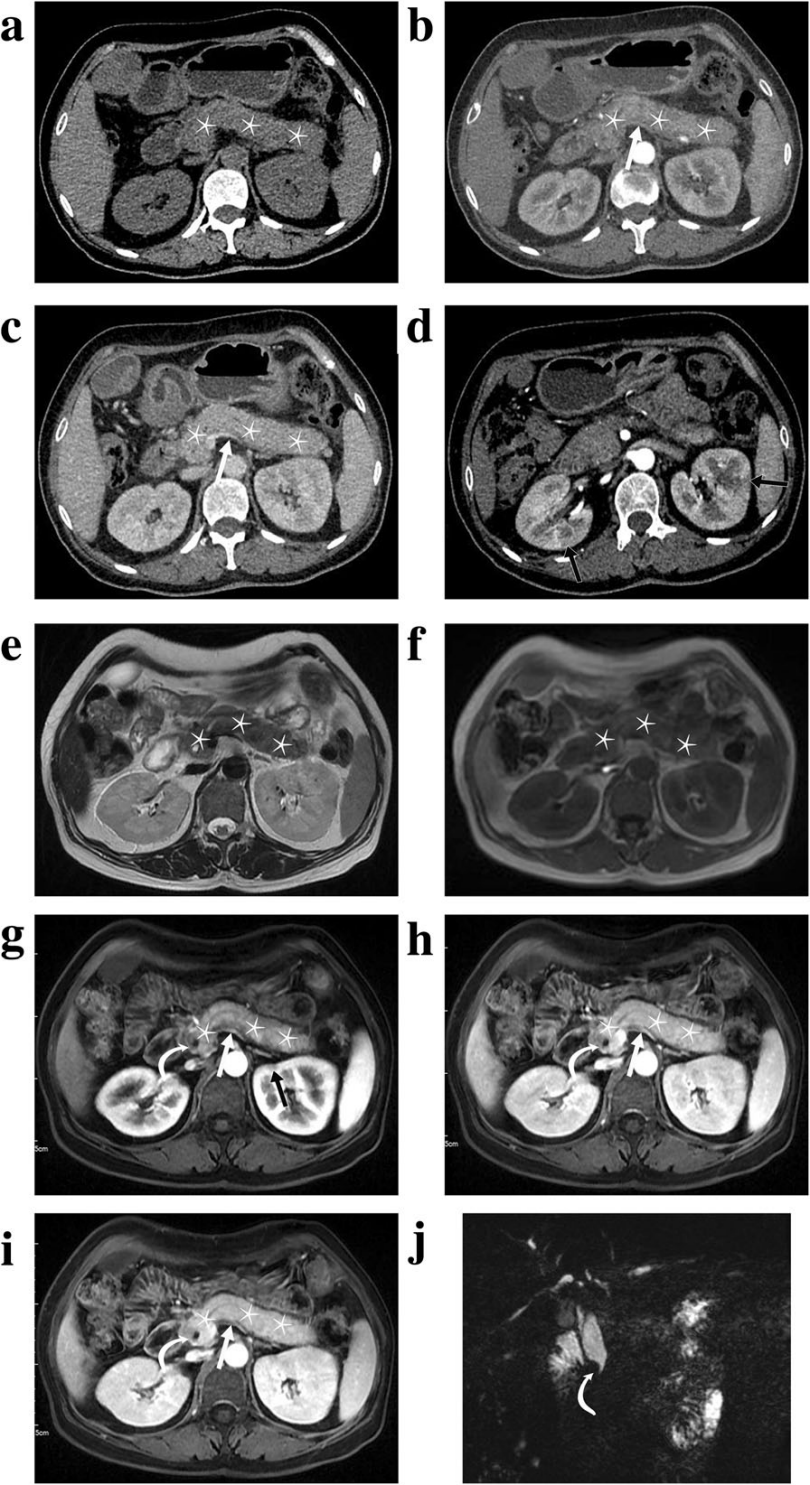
Typical CT and MR imaging appearance of type 1 autoimmune pancreatitis. Axial pre-enhanced (a), arterial (b), venous (c) phase on CT images and axial T2WI (e), T1WI (f), pancreatic (g), portal venous (h) and delayed venous (i) phase on MR imaging showed diffuse pancreatic enlargement with homogenous enhancement (star sign). On MR imaging, it appeared hypointense during the pancratic phase (g) compared with the spleen, and became hyperintense during the portal venous phase (h) compared with the pancreatic phase (g) and isointense during the delayed venous phase (i) compared with the portal venous phase (h). Peripancreatic vessel stricture (straight white arrows) was observed on both CT and MR images (b, c, g, h and i). Extrapancreatic involvement including the common bile duct (curved white arrows on g, h and i) and kidney (straight black arrows on d and g) were noted. The MR cholangiopancreatography (j) showed the stricture of the common bile duct and the dilatation of the upstream extrahepatic duct (curved white arrow)
